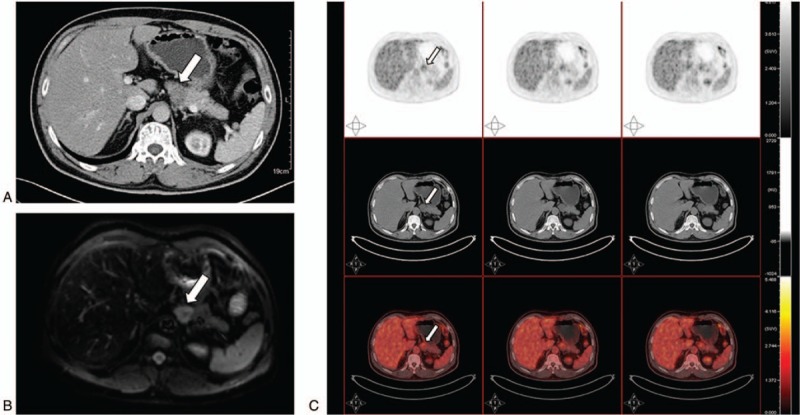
The pancreas was oppressed by the lump located near the fundus of the stomach on contrast-enhanced CT (A, arrow) and the DWI of magnetic resonance image (B, arrow). The tumor could be identified in PET (C, arrow). CT = computed tomography, PET = positron emission tomography.
